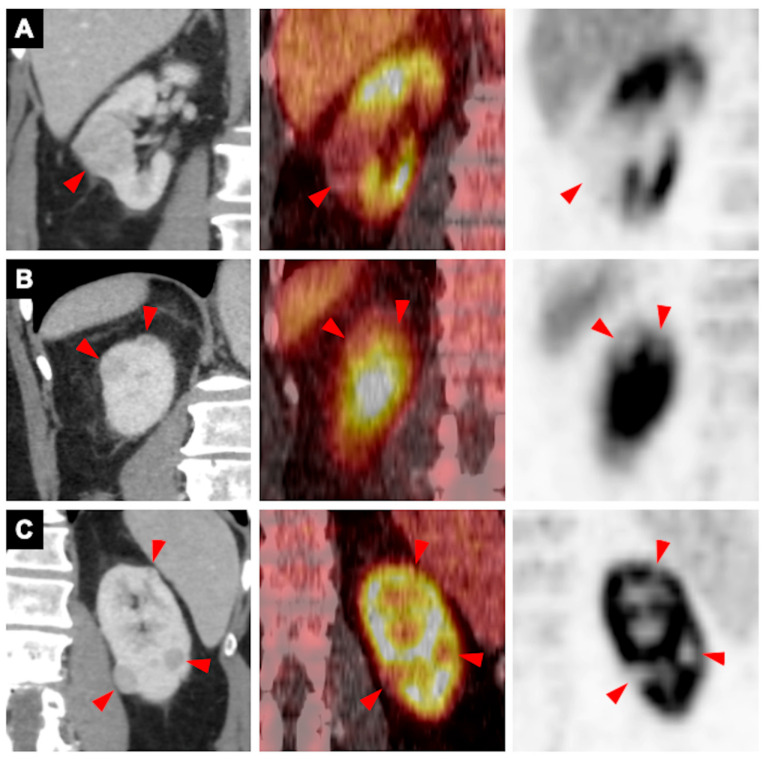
A 76-year-old man with Birt–Hogg–Dubé syndrome presented with multiple indeterminate renal masses. Coronal portal venous phase CT images (left), ^89^Zr-girentuximab PET/CT fused images (middle), and PET images (right) demonstrate multiple solid renal masses, with three in the right kidney (triangles in (A,B)) and three in the left kidney (triangles in (C)). All lesions show lower ^89^Zr-girentuximab uptake compared with the normal renal parenchyma. Biopsy of the largest right renal lesion (triangles in (A)) revealed an oncocytoma. Based on the biopsy and PET/CT findings, a diagnosis of renal oncocytomatosis was established, and the patient was managed with active surveillance.
